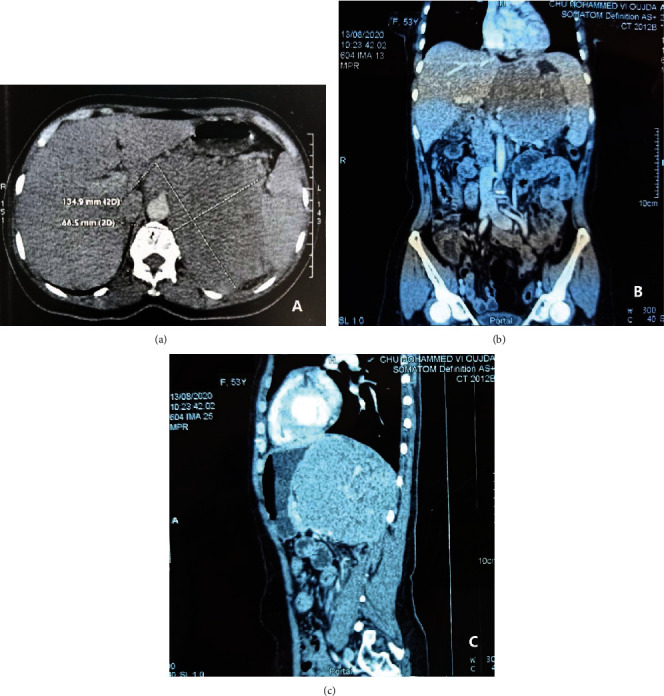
Abdominal CT scan: (a) axial, (b) frontal, and (c) sagittal images show the reports of the locally advanced left adrenal mass of 13.5 cm long axis.
